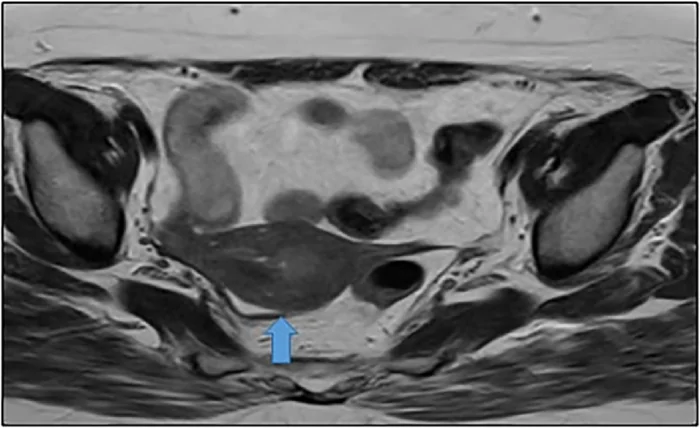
T2-weighted axial magnetic resonance imaging of the pelvis shows a hyperintense polypoidal lesion in the upper endometrium (arrow).
